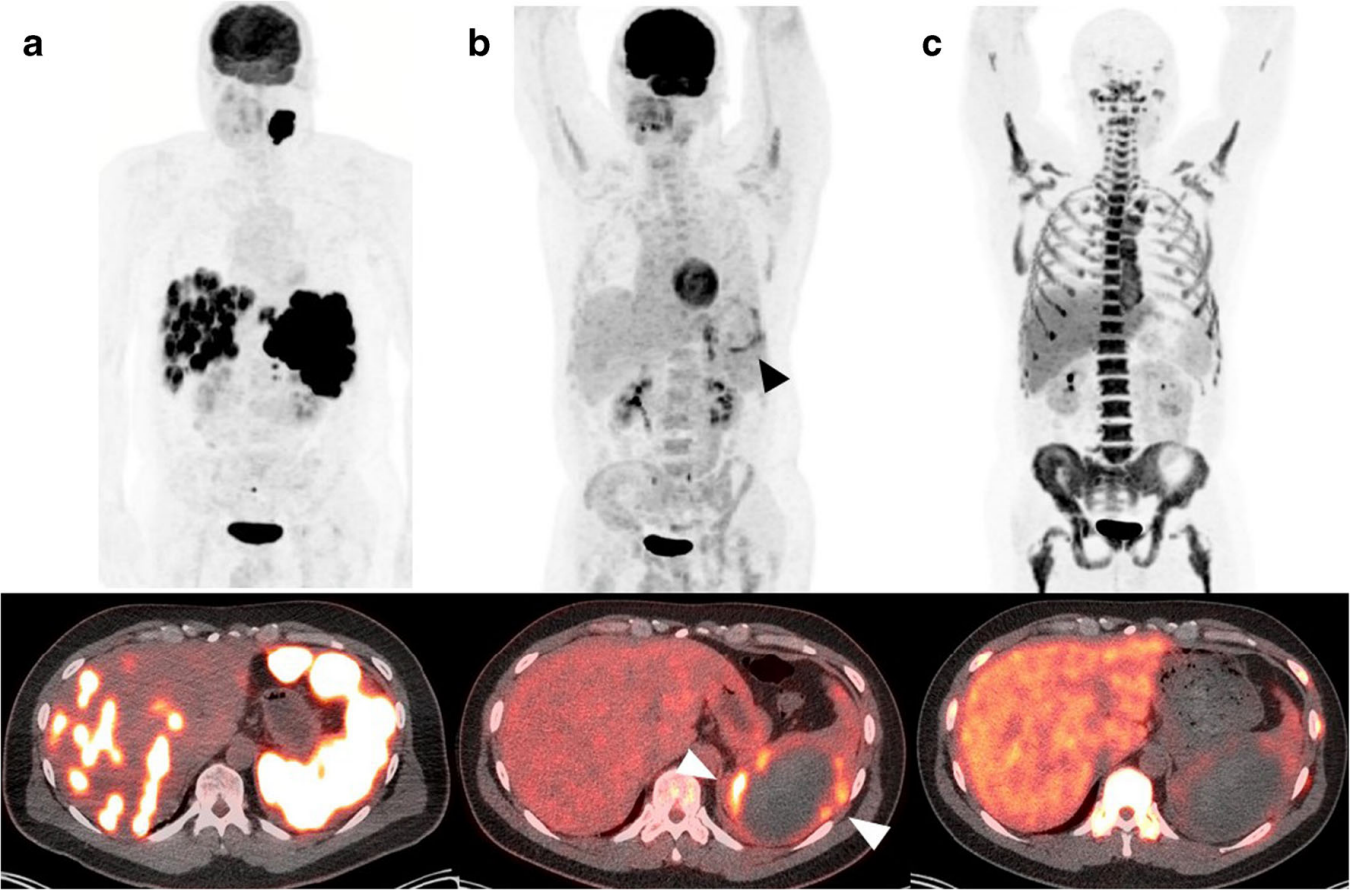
Images in 73-year-old man. a Baseline FDG PET maximum intensity projection (upper row) and axial PET/CT fusion image (lower row) show intense FDG uptake in the liver and spleen. b Interim FDG PET maximum intensity projection (upper row) and axial PET/CT fusion image (lower row) show decreased activity in the liver and spleen, but the lesion remained positive in spleen (arrowhead) due to an area with visually higher than liver FDG uptake in the spleen. c Interim FLT PET image (upper row) and axial PET/CT fusion image (lower row) show no area higher than surrounding area in liver and photopenic tracer uptake in spleen lesion, which was considered negative for disease. This patient remains without evidence of recurrence for 3 years and 2 months from initiation of treatment
